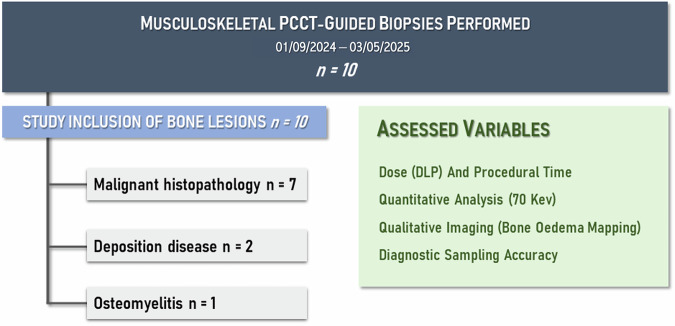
Study the inclusion process of PCCT-guided bone biopsies with exclusion criteria. DLP, Dose-length product; PCCT, Photon-counting computed tomography
